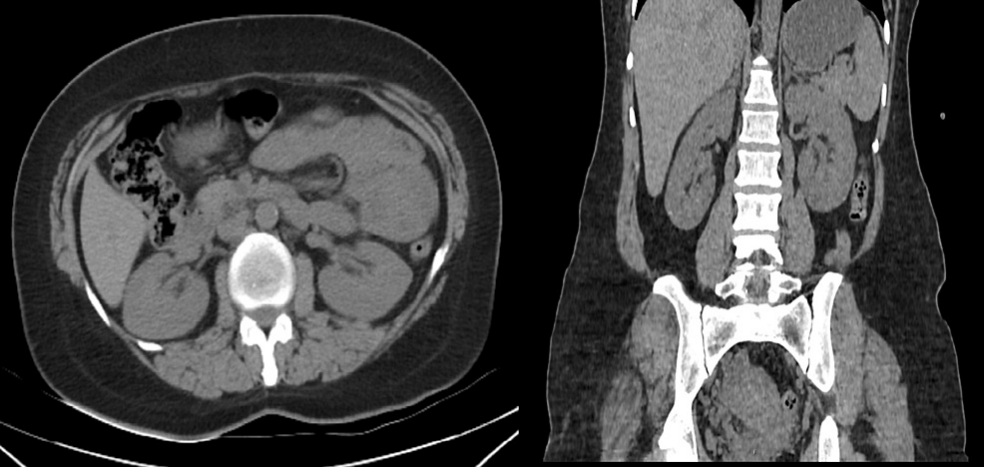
CT abdomen revealed no abnormal findings CT: computed tomography
